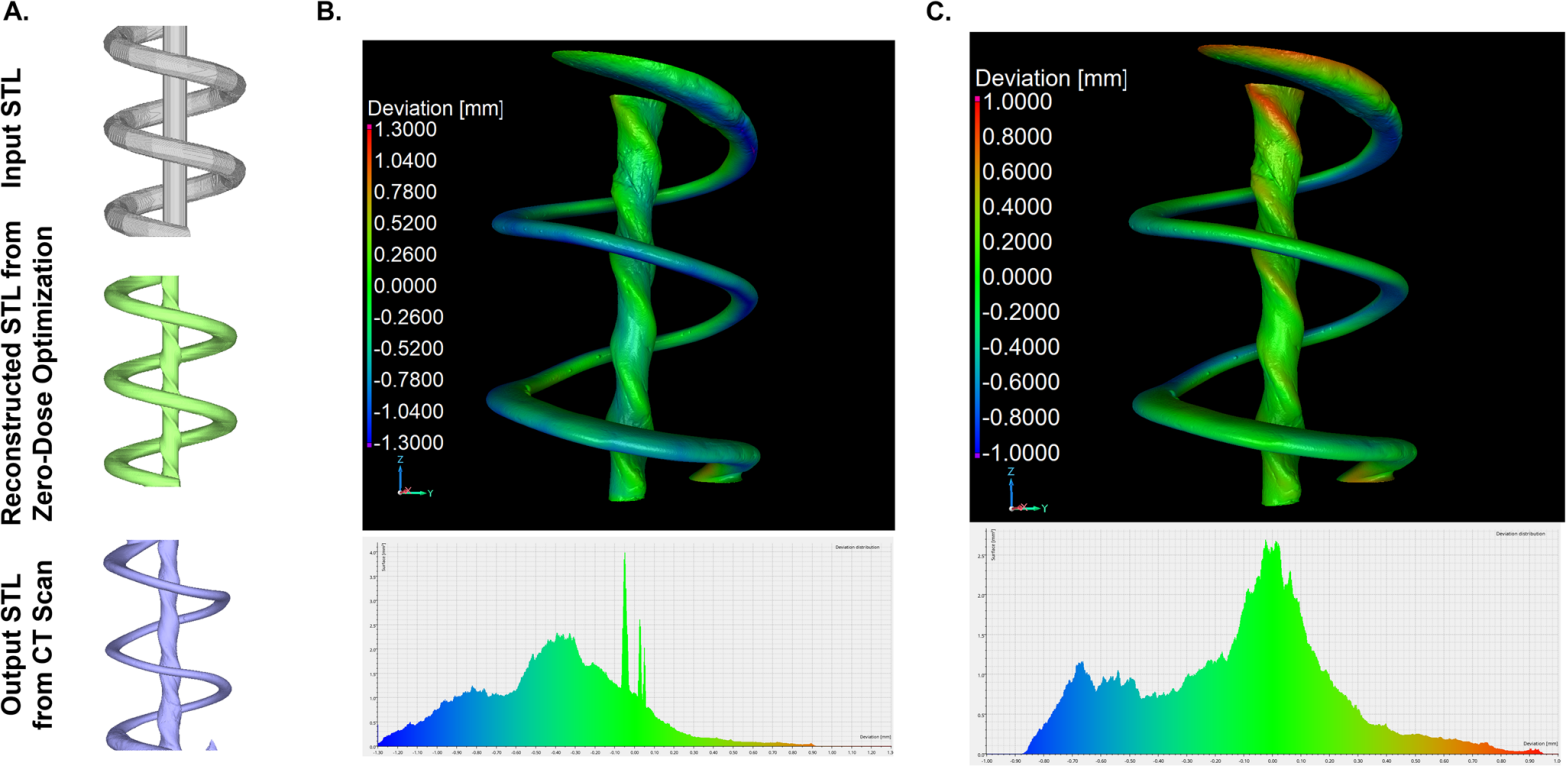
A) From top to bottom, the 1) Target geometry, 2) Reconstructed isosurface from zero‐dose optimization, and 3) Printed geometry generated from the CT scan of the VAM printed millifluidic device. B) Deviation map comparing the CT scan of the printed structure to the input target geometry. C) Deviation map comparing the CT scan of the printed structure to the zero‐dose reconstruction.
